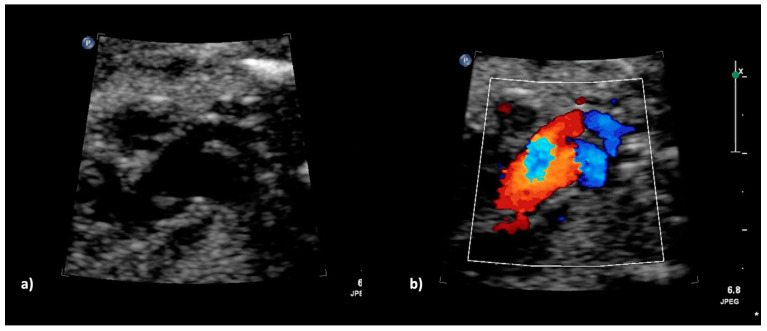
(a,b): Truncus arteriosus type 4 with interrupted aortic arch type b at 23 + 5 weeks. (a) The 2DE shows the ventricular outlet part with the overriding common trunk, the truncal valve as well as the wide truncus pulmonalis and the hypoplastic ascending aorta (pulmonary dominance); (b) The color Doppler mode shows the outflow from both ventricles into the common arterial trunk and further into the pulmonary trunk as well as into the hypoplastic aorta.
